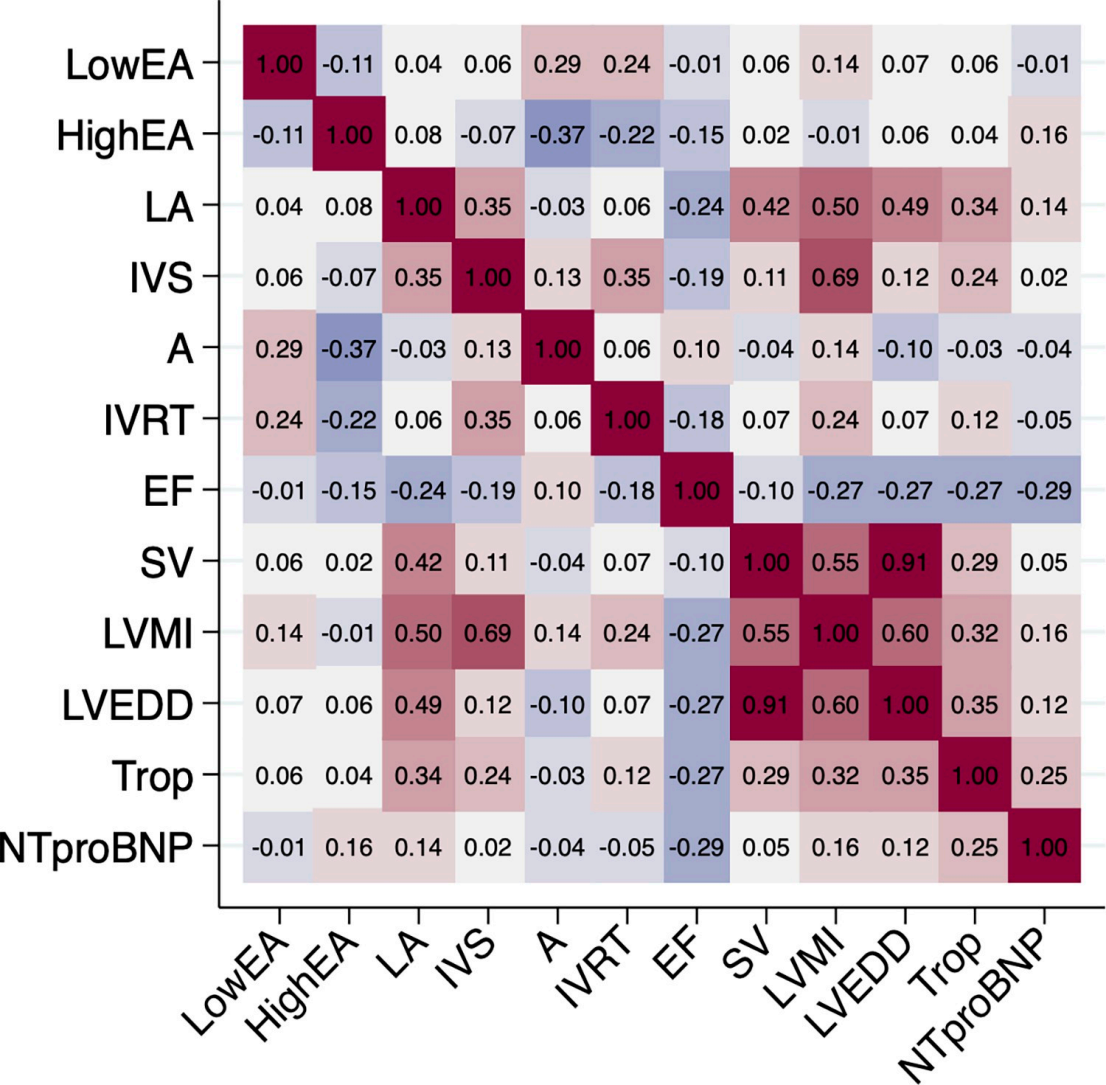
Heatmap detailing the pairwise correlations between the 10 echocardiographic variables and the two cardiac biomarkers, troponin I and NT-proBNP. A, atrial maximal transmitral Doppler velocity; EA, E wave to A wave ratio; EF, ejection fraction; IVRT, interventricular relaxation time; IVS, interventricular septum thickness; LA, left atrial diameter; LVEDD, left ventricular end diastolic diameter; LVMI, left ventricular mass index; NTproBNP, N-terminal-pro hormone B-type natriuretic peptide; SV, stoke volume; Trop, Troponin I.
